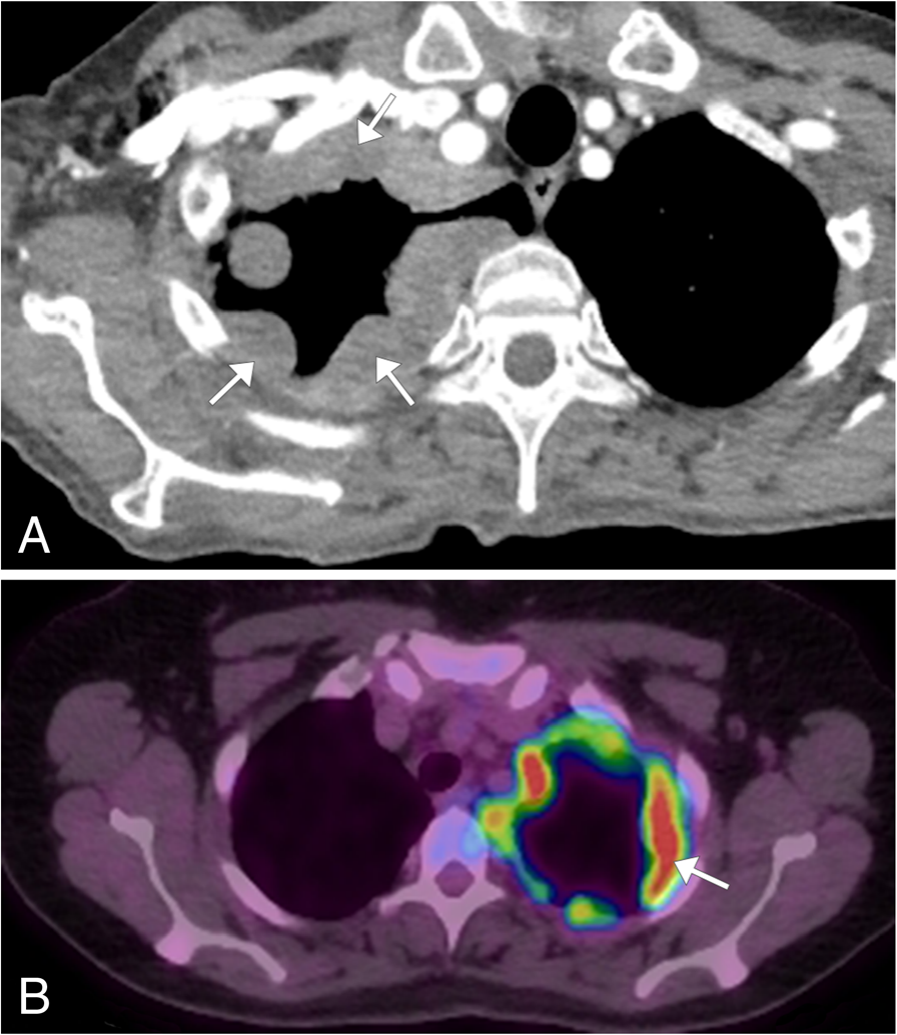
Mesothelioma. a Axial chest CT showing marked nodular thickening of the pleura throughout the right pleural surface (arrows). b Axial FDG-PET/CT image of a companion case showing the hypermetabolic activity of the nodular pleural lesions (arrow) in the setting of biopsy proven mesothelioma
